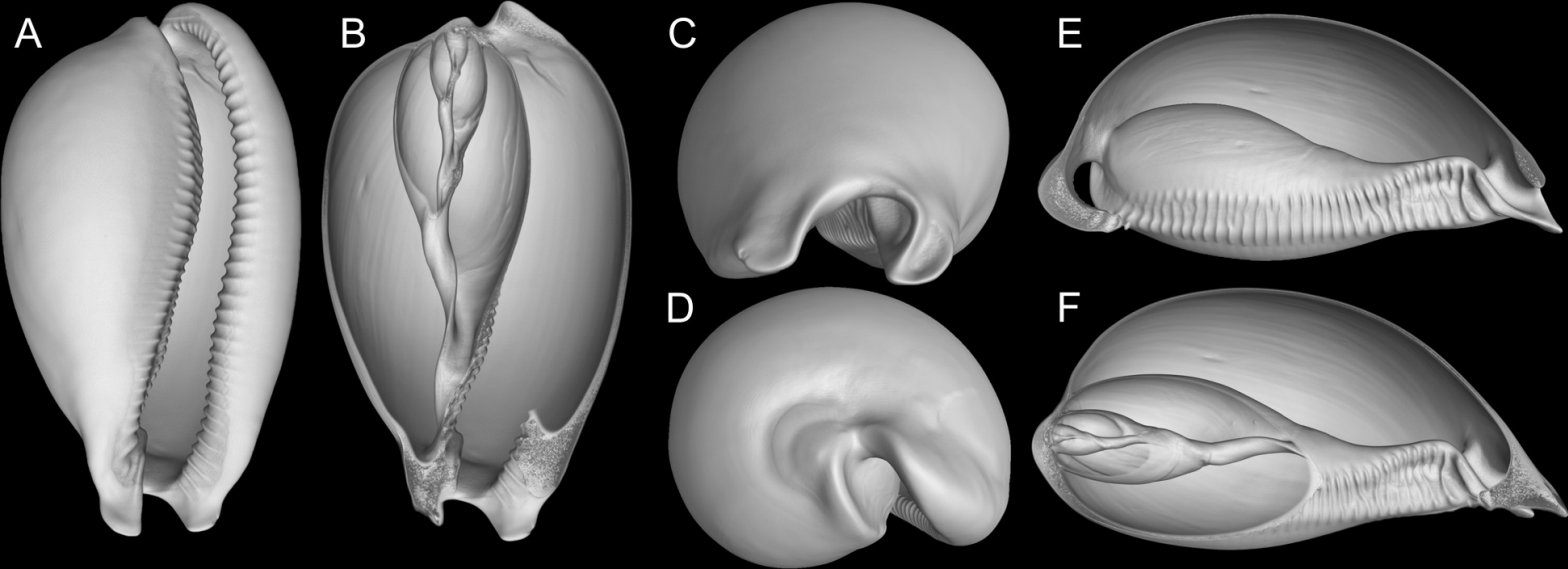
Macrocypraea cervus*, 3D reconstructions of shell (CT-Scan) MZSP 121238 (L 98.5 mm). (A) Frontal view. (B) Same, more ventral portions removed. (C) Anterior view.(D) Posterior view. (E), Right view, right half removed. (F) Same, longitudinal section along columellar axis.
